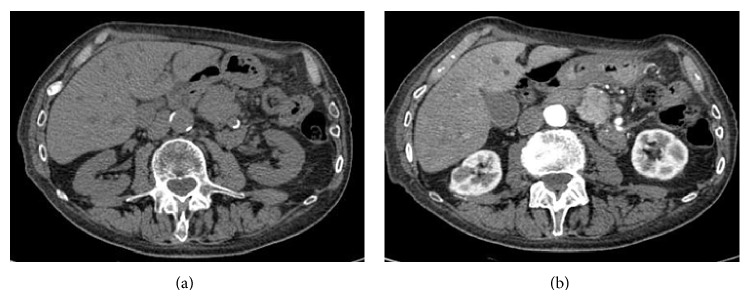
CT evaluation of pancreatic lesion. (a) Nonenhanced CT shows pancreatic body mass isodense to liver parenchyma; (b) pancreatic phase enhanced CT shows hypervascular pancreatic lesion.
